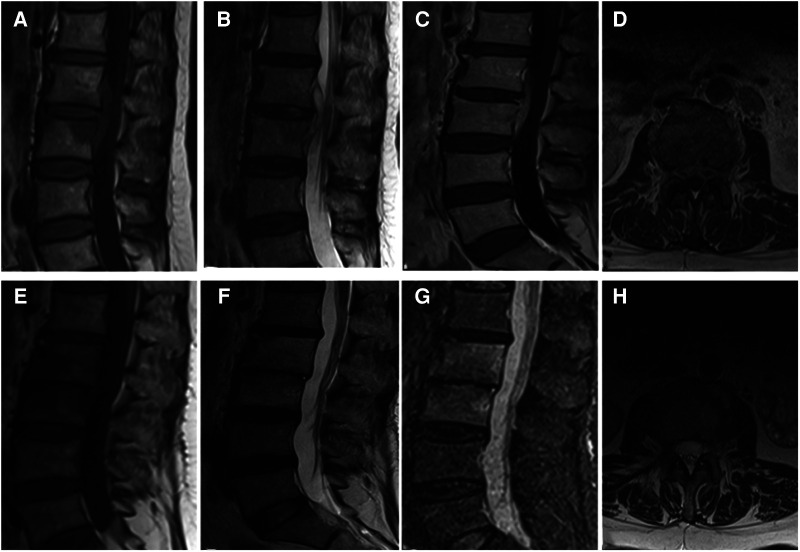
(A–D) It presents the MRI scans of patient No. 9. Sagittal and axial magnetic resonance images with L2-L3 intervertebral infection. (E–H) It presents the postoperative the MRI scans (6 months after surgery) of patient No. 9. MRI shows that the abscess was disappeared without recurrence. (A,E) Sagittal T1WI. (B,F) Sagittal T2WI. (C,G) Sagittal contrast-enhanced image. (D,H) Axial image.
